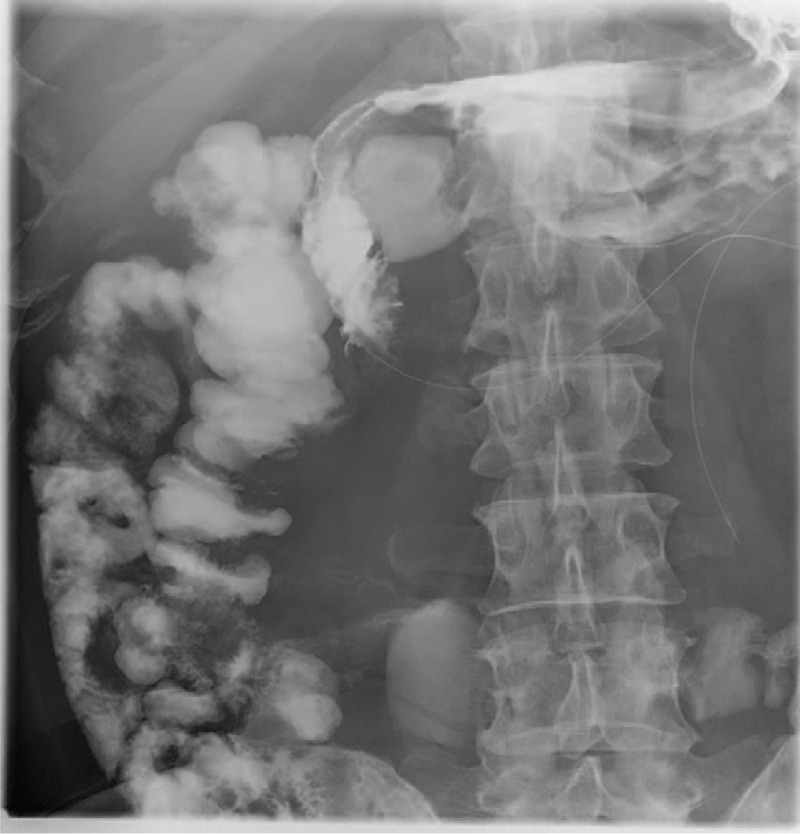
Gastrointestinal radiographs showed that the descending duodenum was narrow.
